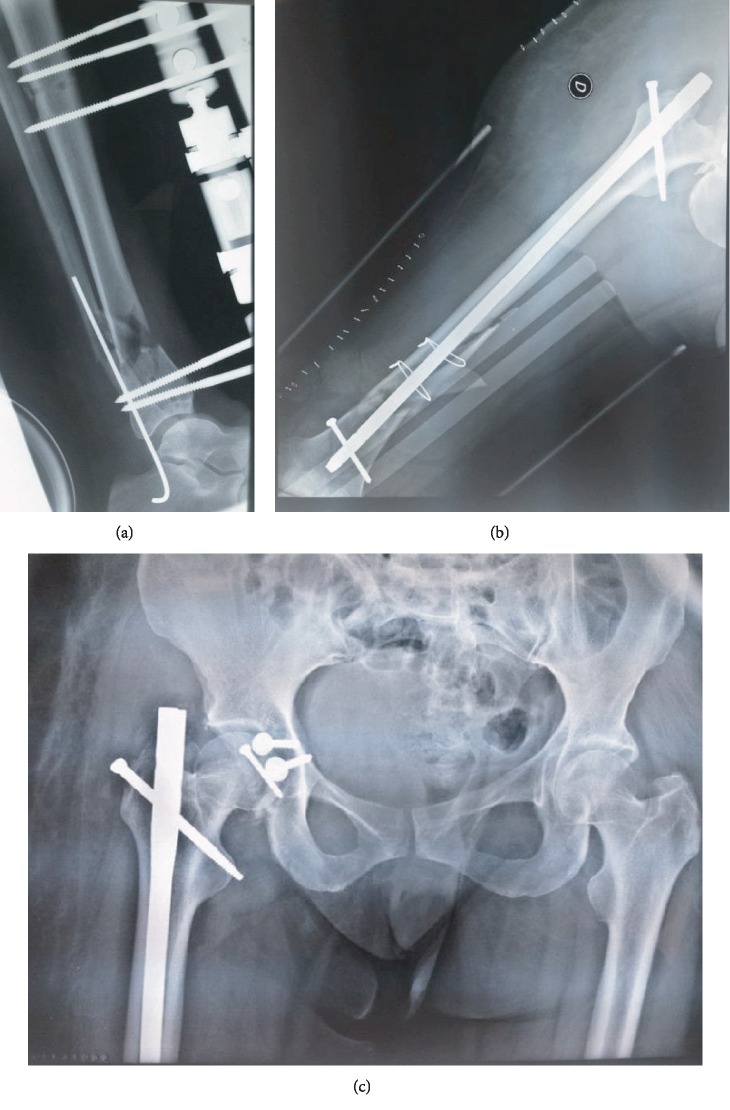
Postoperative radiographs showing fixation of fractures of (a) tibia, (b) shaft of femur, and (c) acetabulum.
